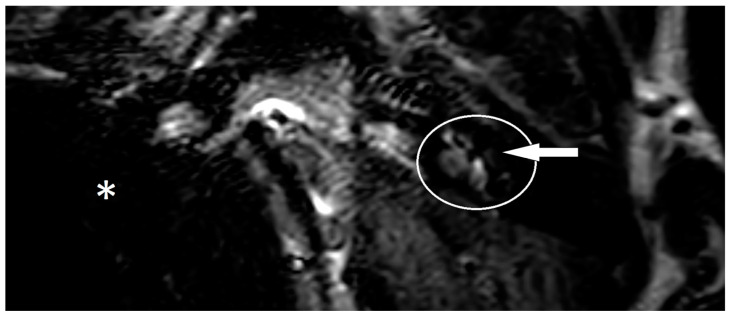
MRI of patient one in a three-dimensional fluid-attenuated inversion recovery (3D-FLAIR) sequence with delayed contrast. The left inner ear is marked with a white circle. The implanted side cannot be seen because of an artifact of the implant. The artifact is marked with a white star. It is possible to mark out a cochlear hydrops grade 1, which is marked with a white arrow.
